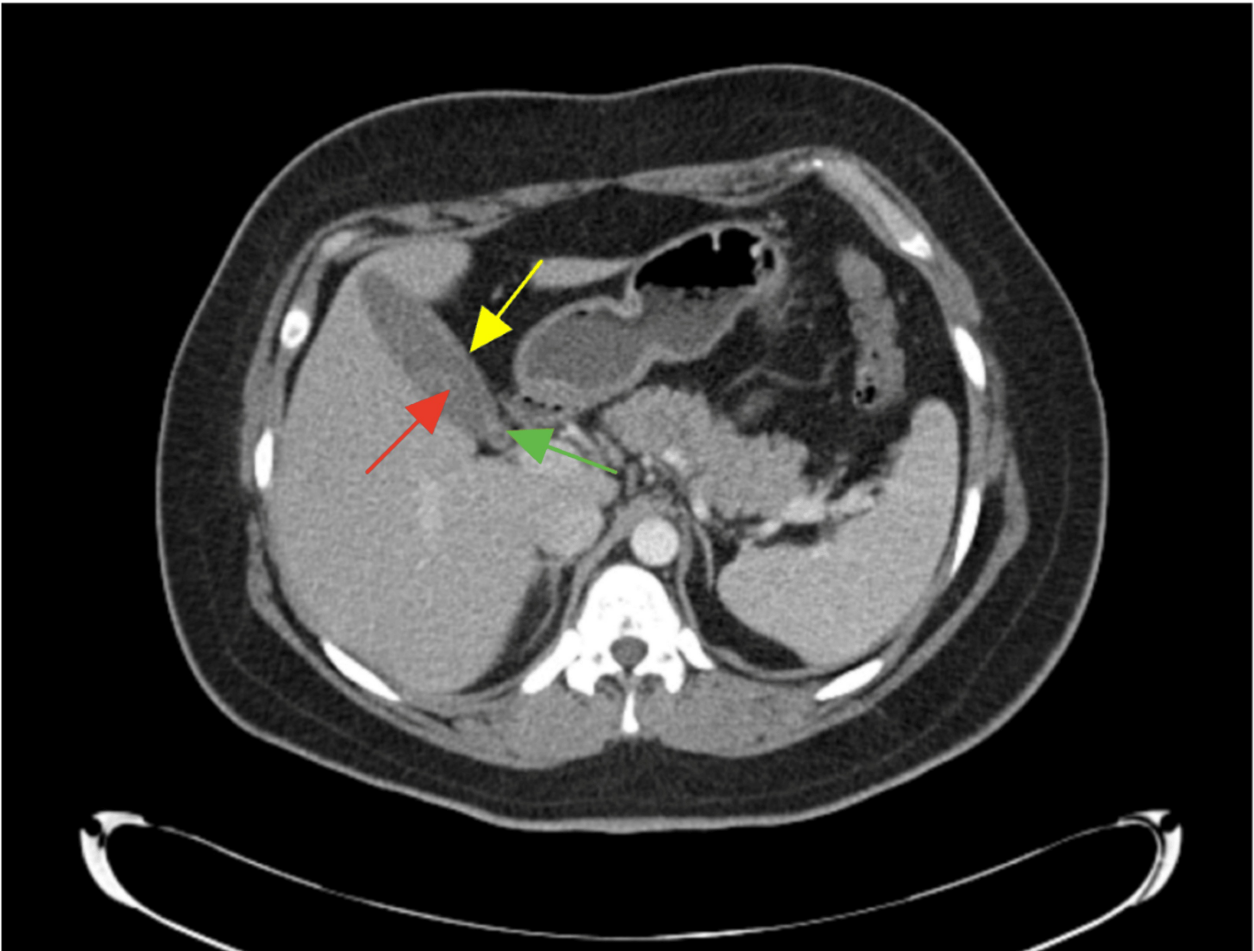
Transverse CT of abdomen on postoperative day 20 Yellow arrow: Mild gallbladder wall thickening; Red arrow: Presence of gallstones; Green arrow: Gallstone evident at the neck of the gallbladder as a possible source of obstruction to outflow of bile secretion
